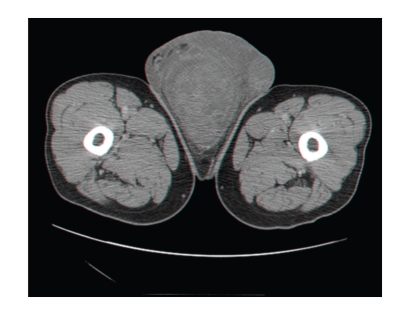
CT scan of the perineum showing a median scrotal mass.
